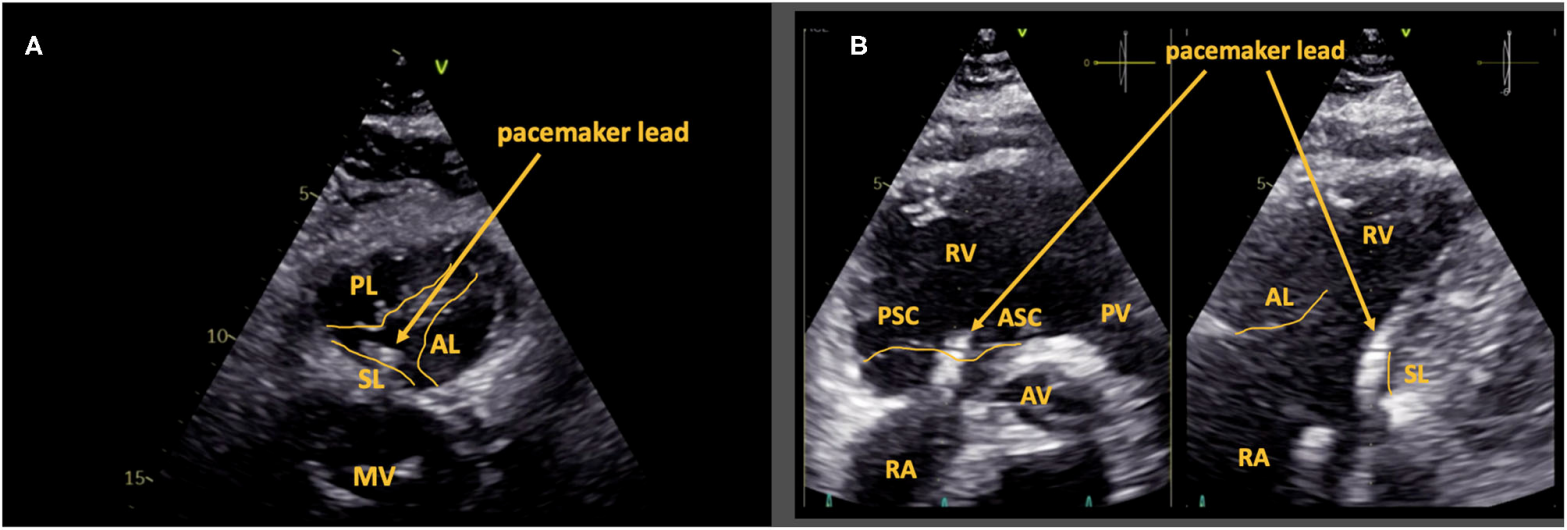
Case 1: Septal leaflet impingement by pacemaker lead. Central lead position with impingement of the septal leaflet is shown *via* subcostal en face view of the tricuspid valve (A) and *via* the commissural view in the parasternal short axis (B; left side) and the corresponding 90° view of the septal and the anterior leaflet (B; right side). AL, anterior leaflet; PL, posterior leaflet; SL, septal leaflet; RA, right atrium; RV, right ventricle; PSC, postero-septal commissure; ASC, antero-septal commissure; MV, mitral valve; PV, pulmonary valve; AV, aortic valve.
